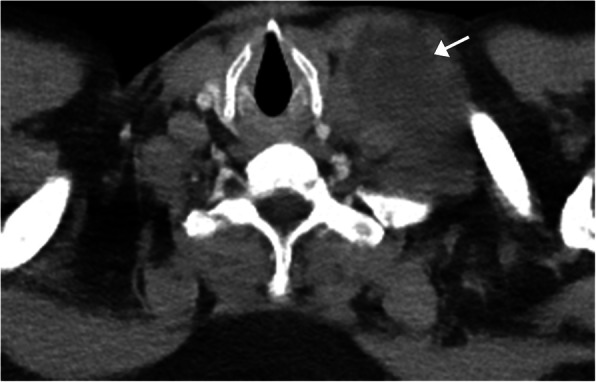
Metastatic lymph node. Axial contrast enhanced chest CT image shows a large ill-defined left supraclavicular lymph node with peripheral soft tissue attenuation and area of central hypoattenuation, likely representing central necrosis (arrow). This lymph node was consistent with metastatic disease in the setting of gastric carcinoma, a classic example of a Virchow’s node
